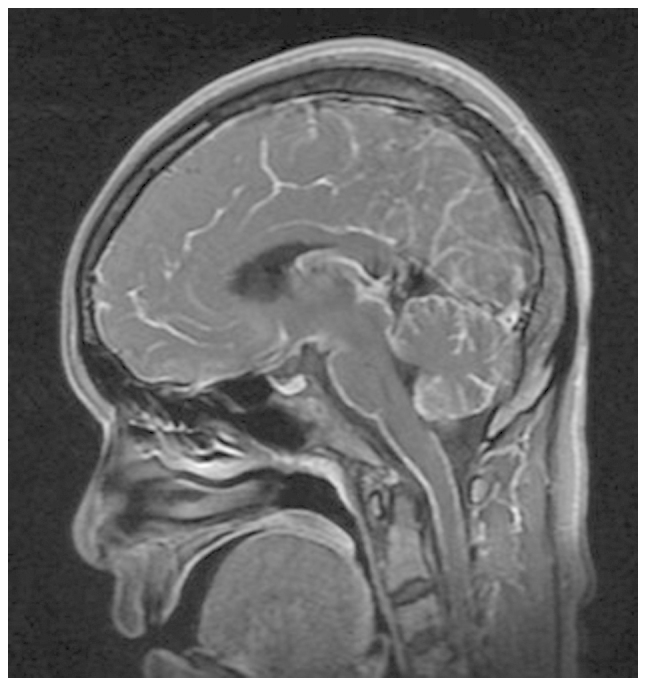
Case 6. Magnetic resonance imaging. Enhanced T1 sagittal view demonstrated enhanced diffused meninges.
